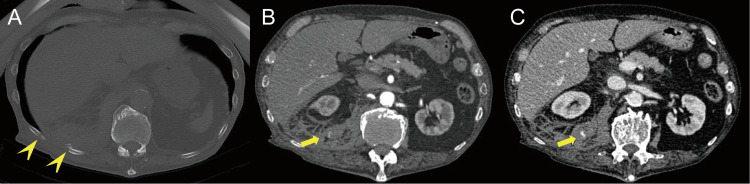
Pretreatment imaging. (A) Non-contrast CT scan with a bone window, (B) 30 seconds after injection of contrast medium, and (C) 80 seconds after injection of contrast medium. Axial non-contrast CT images show two fractures of the right 11th and 12th ribs (arrowhead). Axial contrast-enhanced CT showing a retroperitoneal hematoma with active extravasation (arrow).
